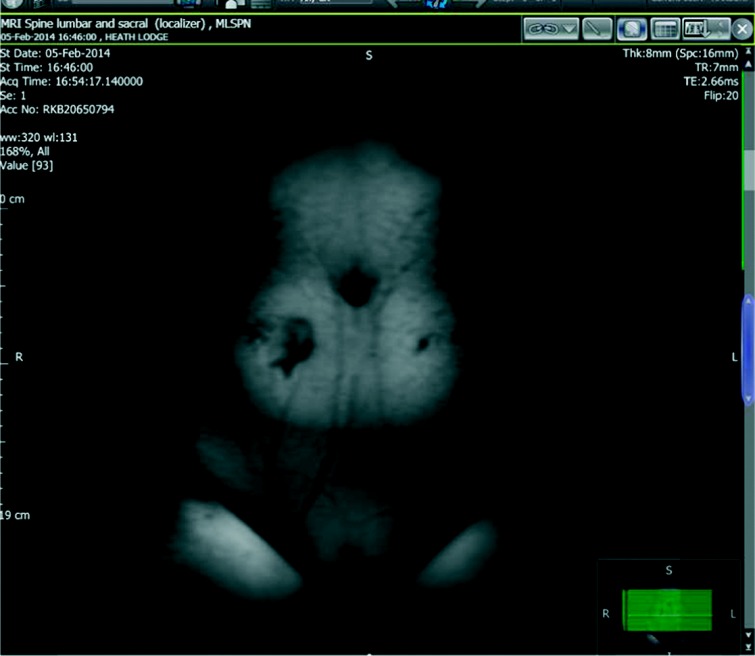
MRI image of the subcutaneous fat necrosis.
